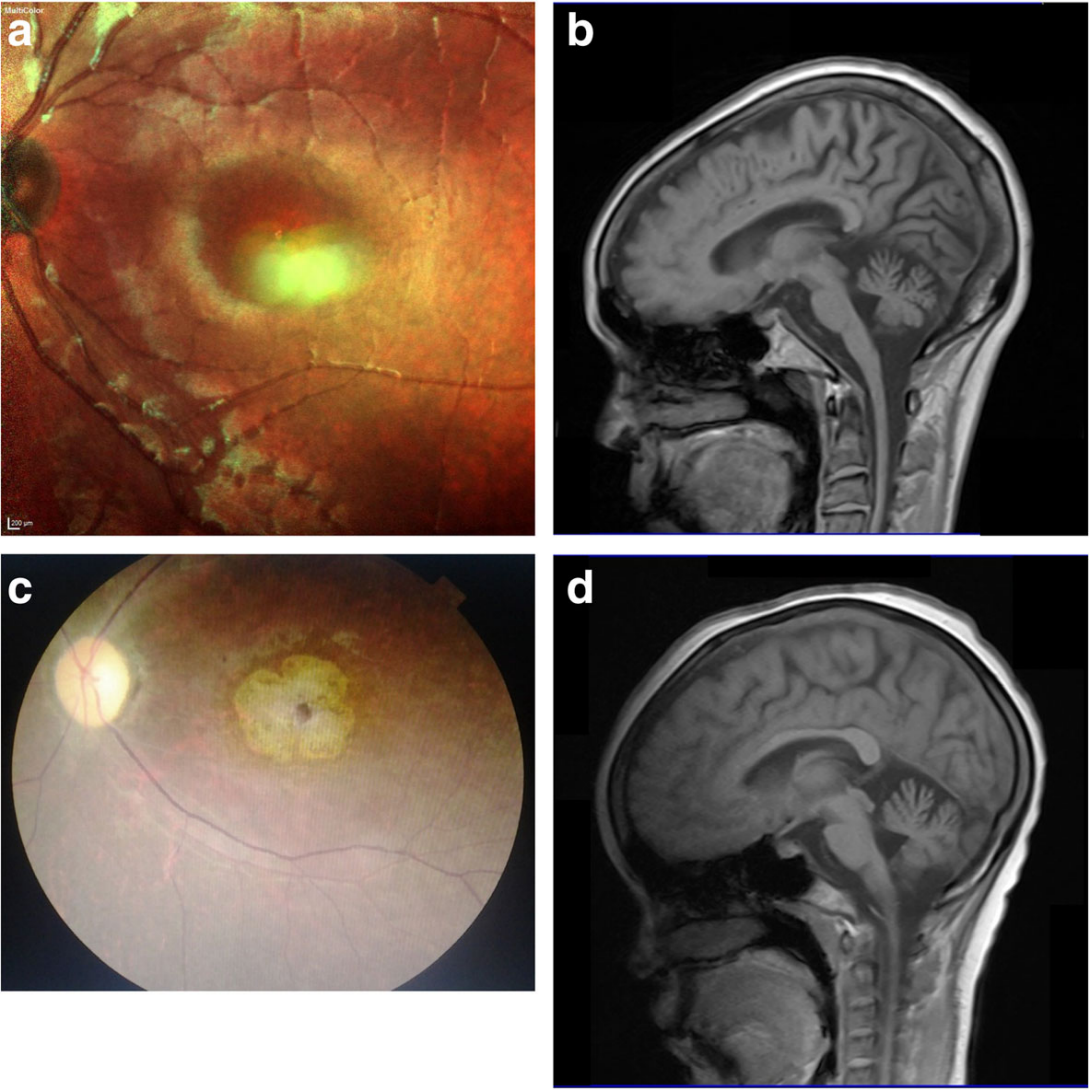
a Photograph of the right fundus of the proband’s brother of Family 1 (iii.12), showing macular degeneration. b T2-weighted MRI brain of patient ii.10 of Family 1 demonstrating diffuse cerebral and cerebellar atrophy, and mild brainstem atrophy. c Photograph of the right fundus of individual iv.10 of Family 3 showing macular degeneration and optic atrophy. d T2-weighted MRI brain of individual iv.10 of Family 3 showing mild cerebral and cerebellar atrophy
